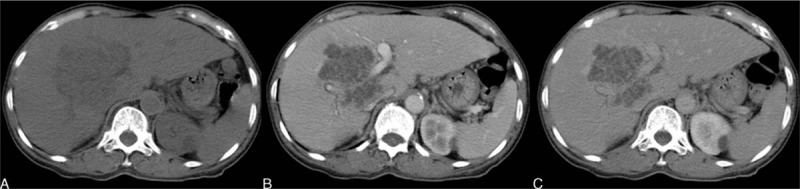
A 71-year-old woman with an inflammatory pseudotumor (IPT). A, Precontrast CT image reveals a relatively hypodense mass. B, Contrast injection revealed a lack of arterial enhancement in the tumor mass. C, Subtle later peripheral and septated enhancement in the delayed phase. CT = computed tomography.
